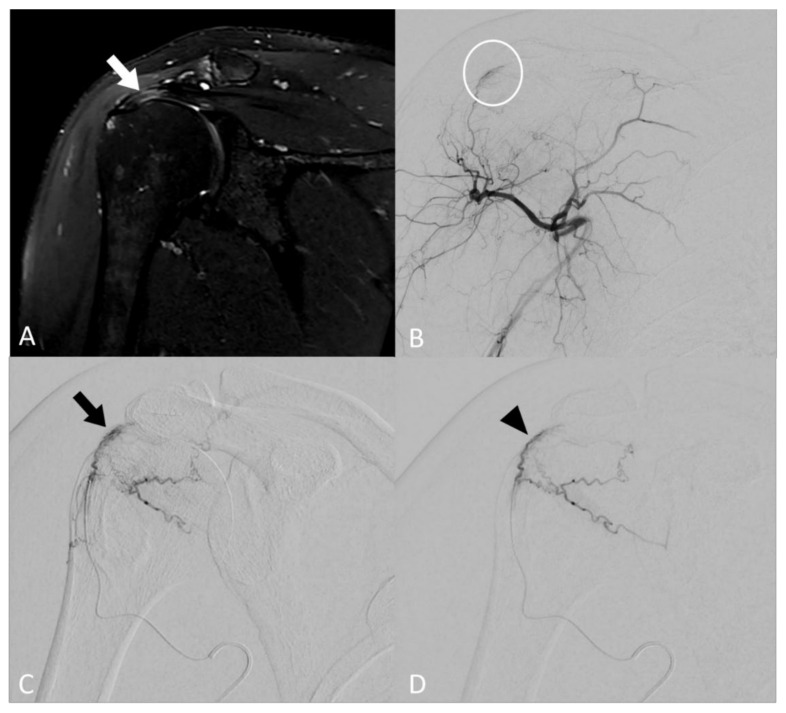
Image of a 46-year-old patient with rotator cuff tendinobursitis refractory to conventional treatment. (A) On baseline coronal T2 fat-sat MR imaging, injury appears as a hypersignal of supraspinatus tendon (white arrow). (B) The digital subtracted angiography (DSA) from the ostium of the posterior circumflex humeral artery confirmed pathological neovessel development in the area supplying the supraspinatus tendon (white circle). (C) DSA after selective microcatheterization of the pathological branch confirmed the “tumor-like” blush (black arrow). (D) DSA after embolization using microspheres demonstrated a decrease in the vascular blush (black arrowhead) that persisted, considering that the collaterals may be at risk of non-targeted embolization complications.
